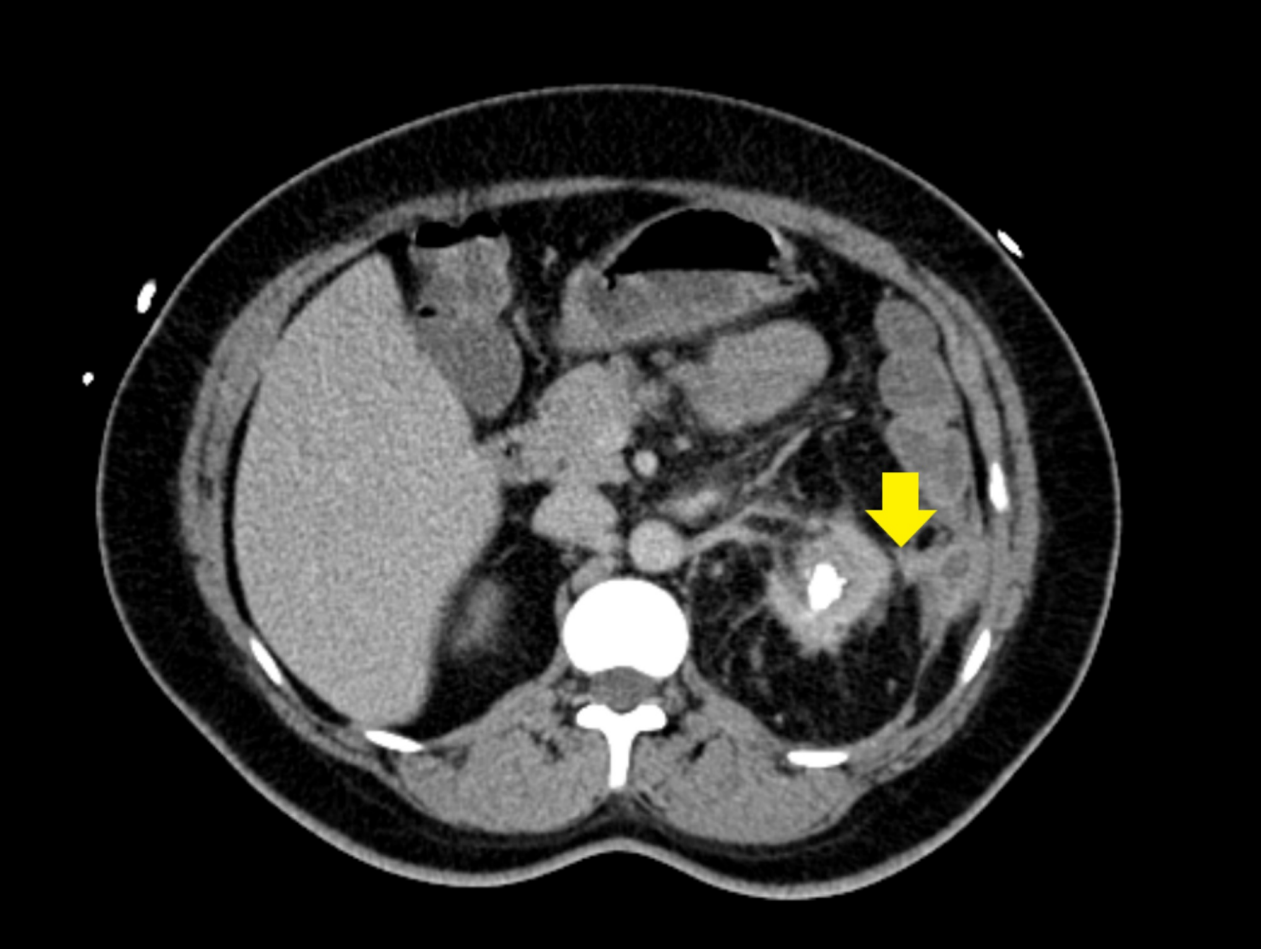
Computed tomography scan of the abdomen and pelvis showing the renocolic fistula
